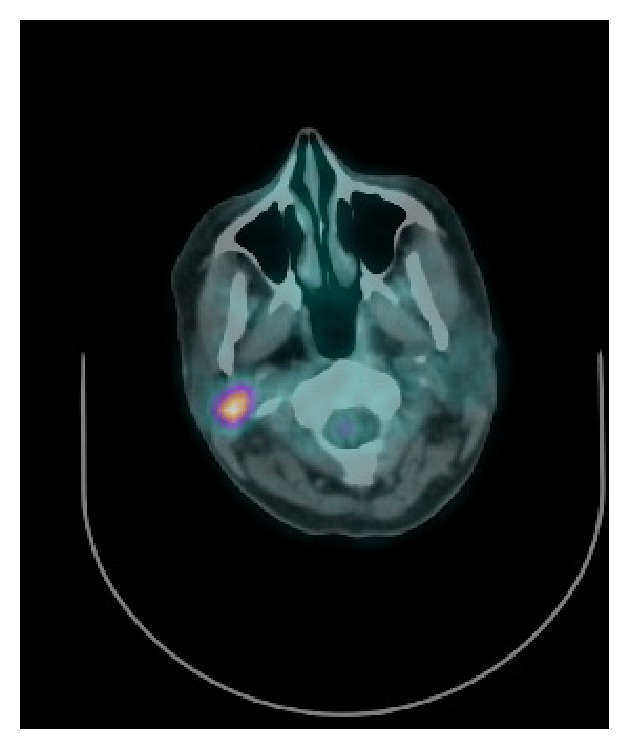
Pet CT showing accumulation of 18-FDG in the right parotid gland.
